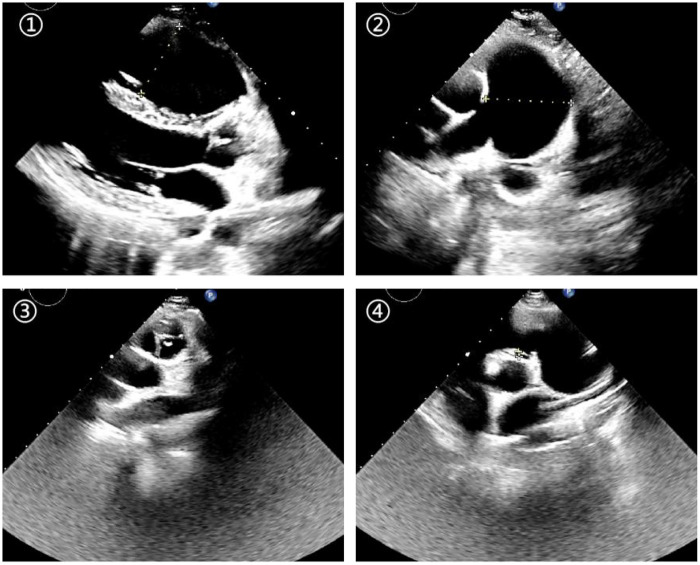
Echocardiography showing right ventricular dilatation (①), pulmonary artery dilatation (②), anterior and right pulmonary valve leaflets (③), and downward displacement of the left pulmonary valve leaflet (④).
